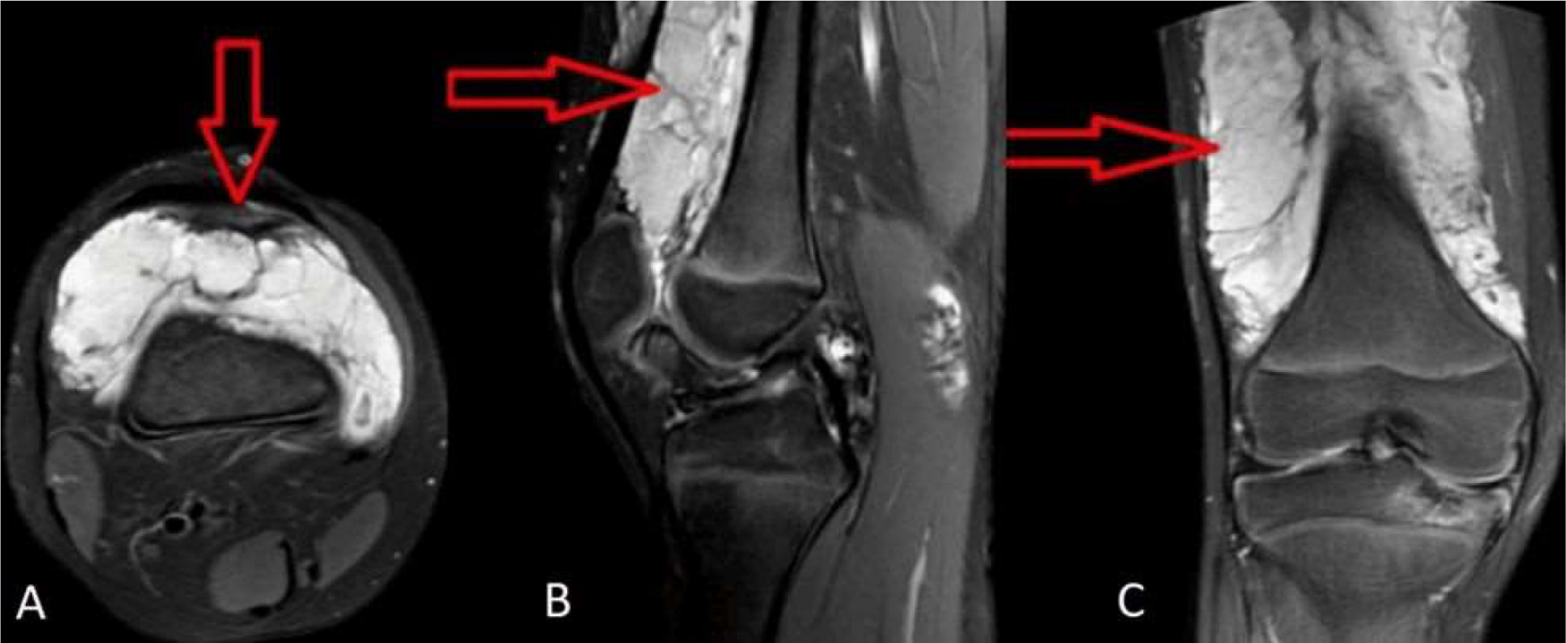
Magnetic Resonance (MRI) showing multiple, dilated, tortuous serpentine Proton Density Fat Saturated (PDFS) hyperintensity along vastus lateralis muscle with hypertrophy of the muscle (red arrow). A-MRI PDFS axial section, B-MRI PDFS sagittal section C-MRI PDFS coronal section.
